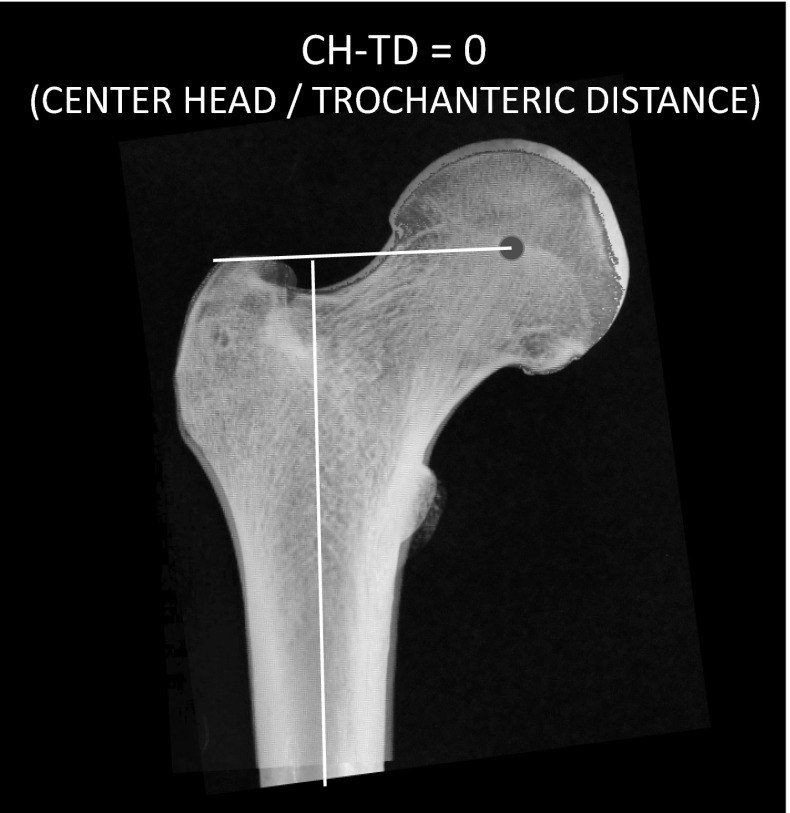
The center of femoral head to trochanteric tip vertical offset is normally zero. This affords maximum efficiency for the abductors. In preadolescent children, this distance is not measurable on plain radiographs because the tip of the trochanter is not ossified. In our series, transtrochanteric rod insertion did not result in any observed change in the center head/trochanteric distance (CH–TD)
